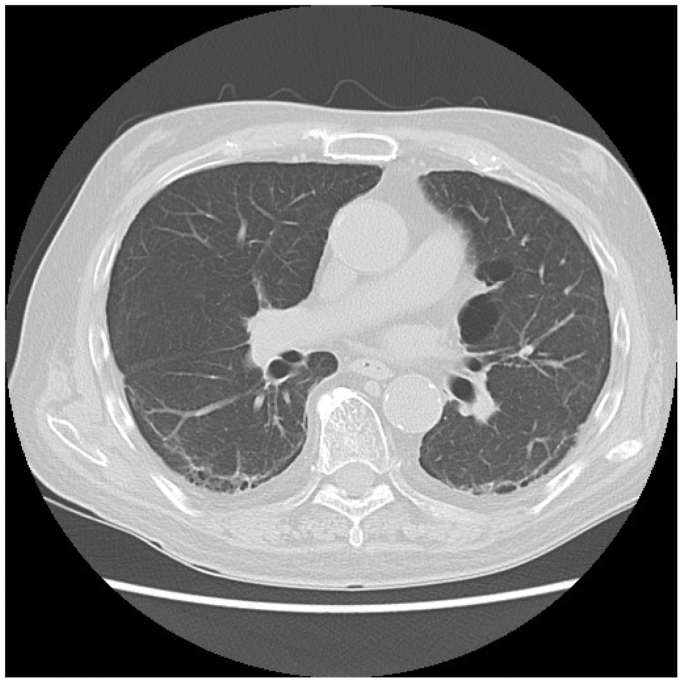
Follow-up chest computed tomography (CT) demonstrating gradual resolution of the previously noted bilateral ground-glass opacities.
